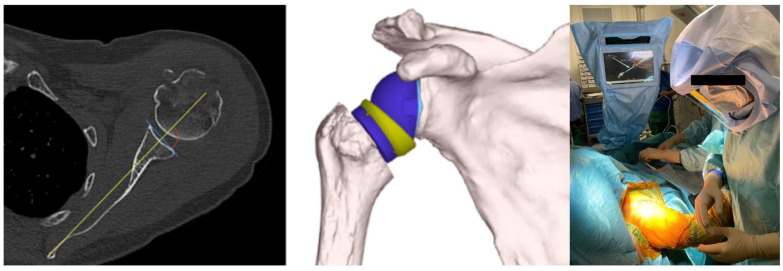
The surgical procedure for reverse shoulder arthroplasty with GPS navigation involves a preoperative CT scan, planning, and intraoperative navigation.
